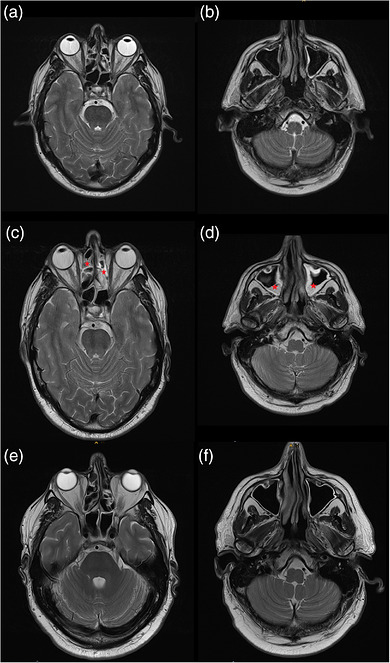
Axial T2‐weighted MRI images of Patient 2 showing (a, b) normal baseline imaging before ICI therapy; (c, d) pronounced mucosal thickening of the ethmoid sinuses with early involvement of the maxillary sinuses under ICI therapy (red asterisks); (e, f) substantial improvement following systemic corticosteroid treatment.
